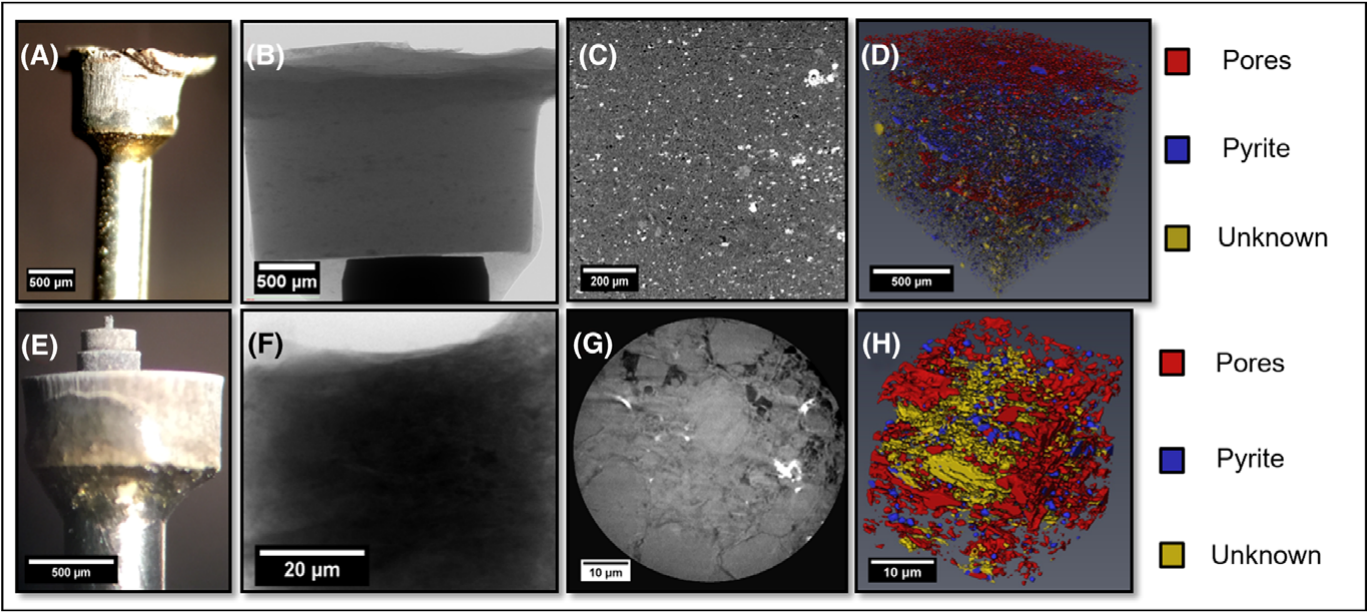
(A) Optical image of coarse pillar for micro‐CT. (B) Corresponding radiograph. (C) Raw orthoslice displaying four phases. (D) 3D reconstruction showing the extra segmentable phase. (E) Optical image of fine pillar for nano‐CT. (F) Corresponding radiograph. (G) Particulate microstructure evident from raw slice. (H) 3D reconstruction of clay particles at high resolution.
